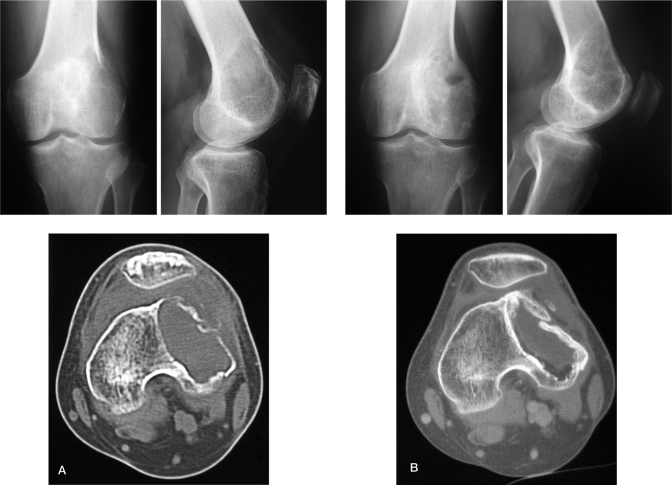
A 34-year-old male with a giant cell tumor in the left femur. A. Computed tomography showed a thin wall around the cavity 1 month after the curettage. B. 1 year postoperatively, bone formation was excellent and the cortex of the cavity was thicker than the normal cortex of the medial condyle.
